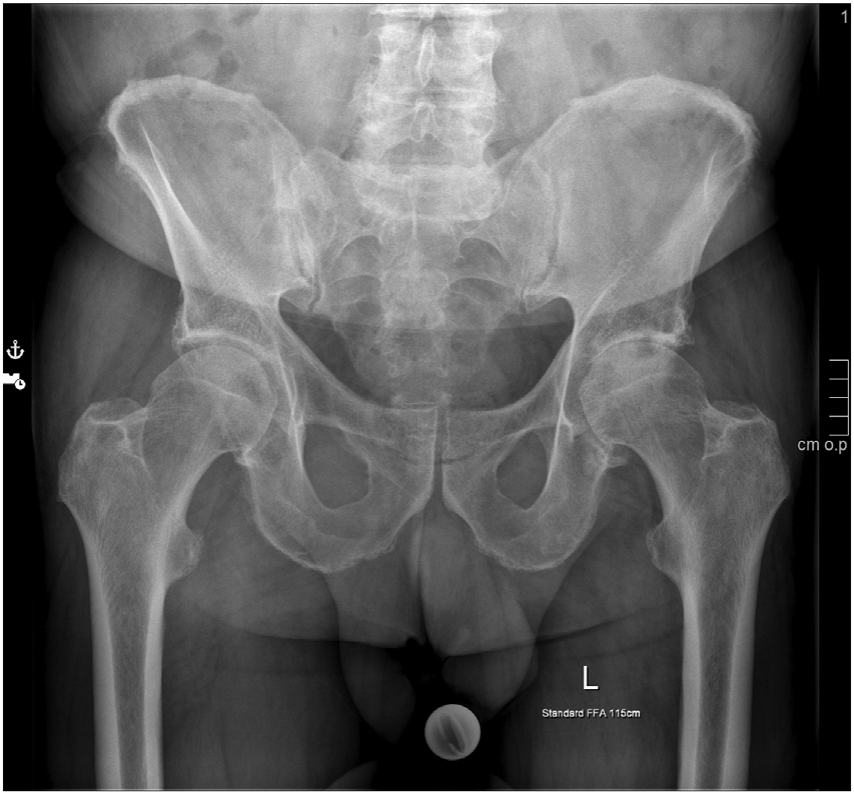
Preoperative radiograph of the patient with hip osteoarthritis (Kellgren-Lawrence Stage 3).
